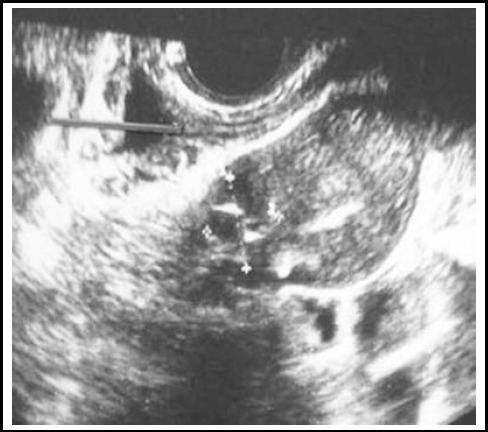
Transvaginal ultrasound image (coronal plane) showed a gestational sac in the left uterine horn.
